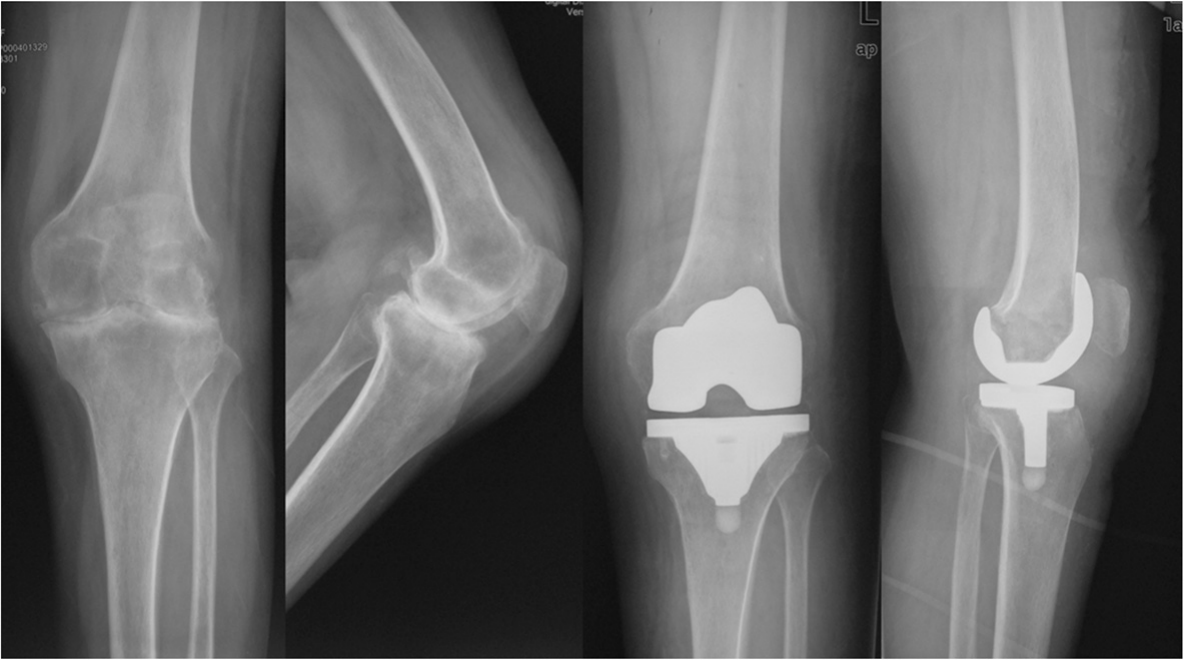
Plain anteroposterior and lateral radiographs of a 43-year-old female which reveal joint destruction. This case is a 43-year-old female patient with RA with flexion contractures deformity. After TKA on the right knee, the 27° flexion contracture was completely corrected postoperatively.
